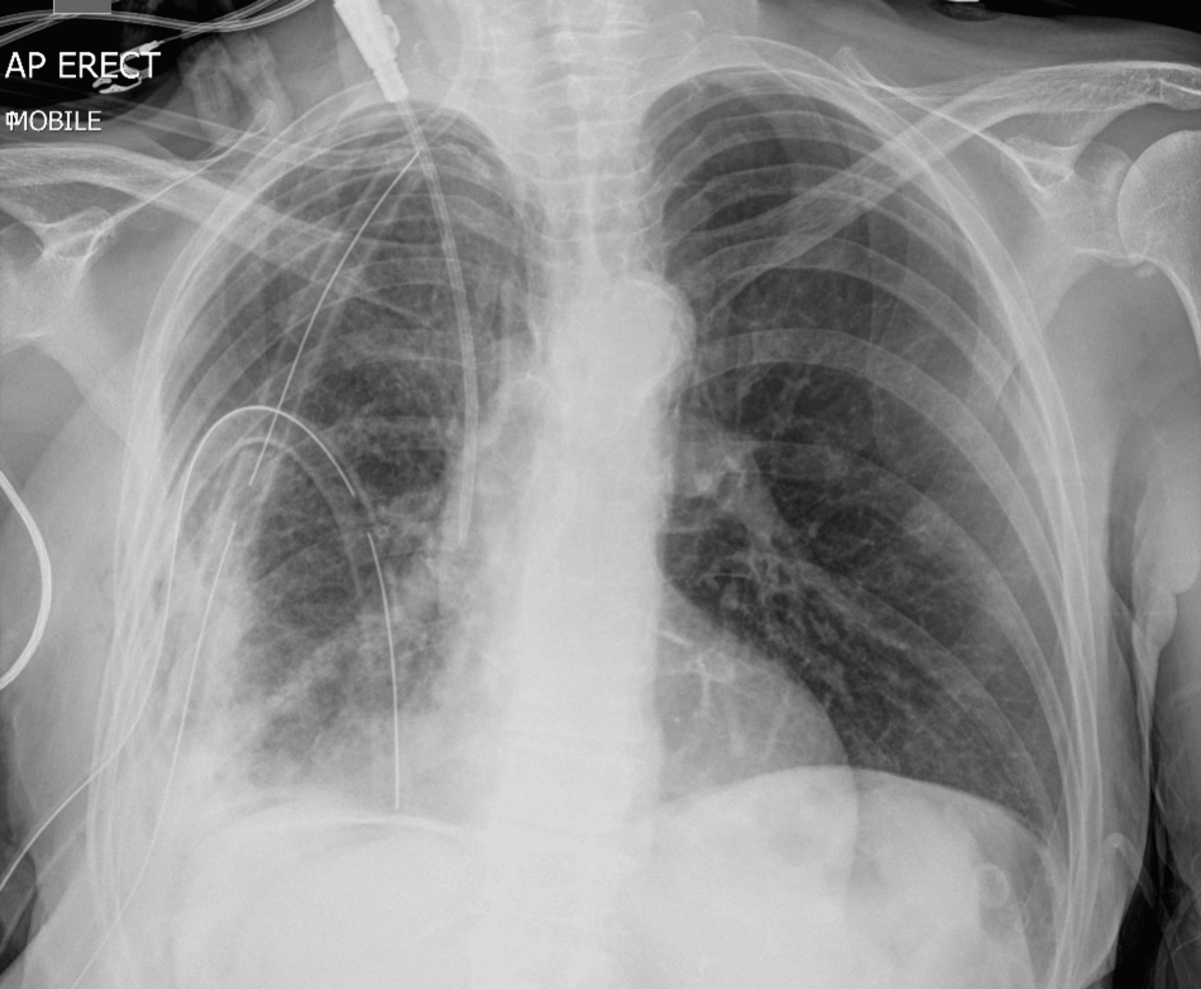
Chest X-ray following VATS. Note the presence of two large bore drains in the apical and basal regions of the right lung VATS: video-assisted thoracic surgery
